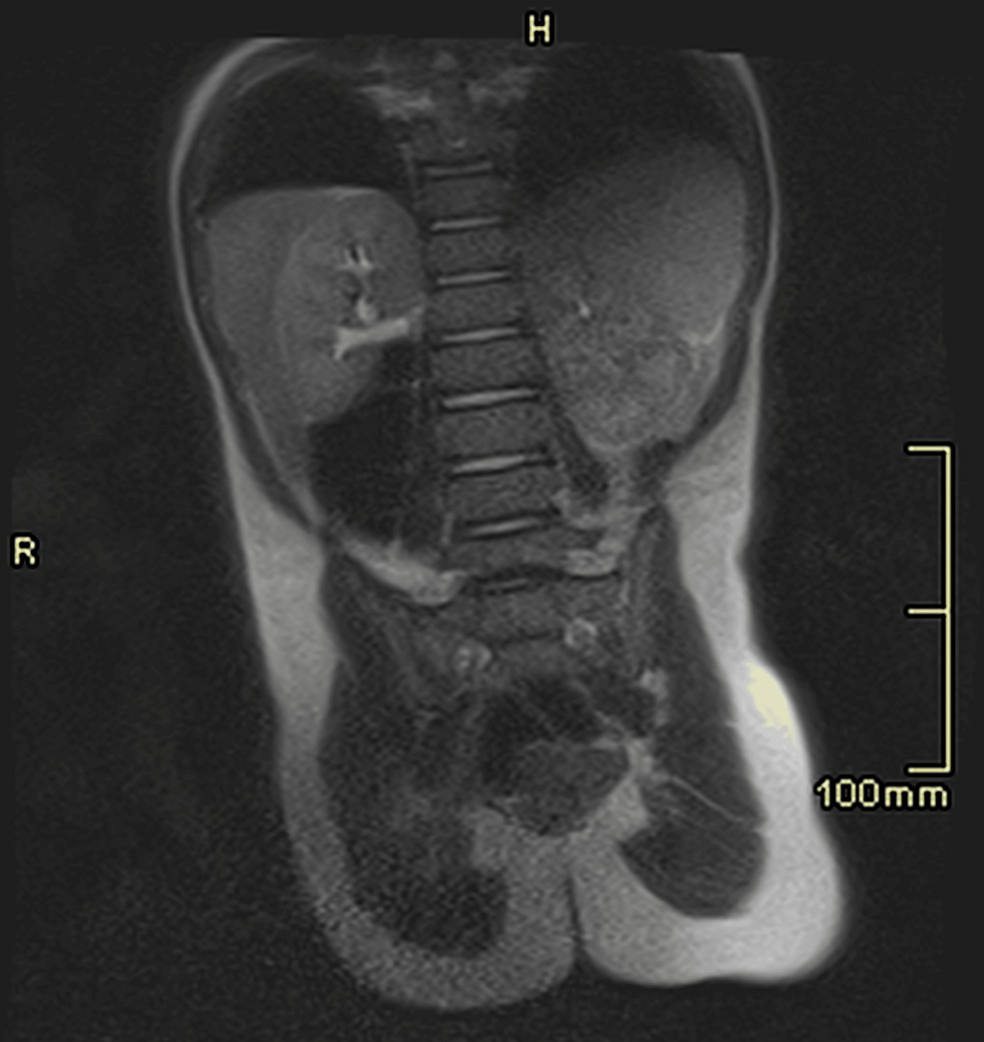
Another 1.5 T MRI T2-weighted coronal imaging of the same child’s abdomen demonstrating the quality of coronal imaging using the abdomen-to-abdomen method.
